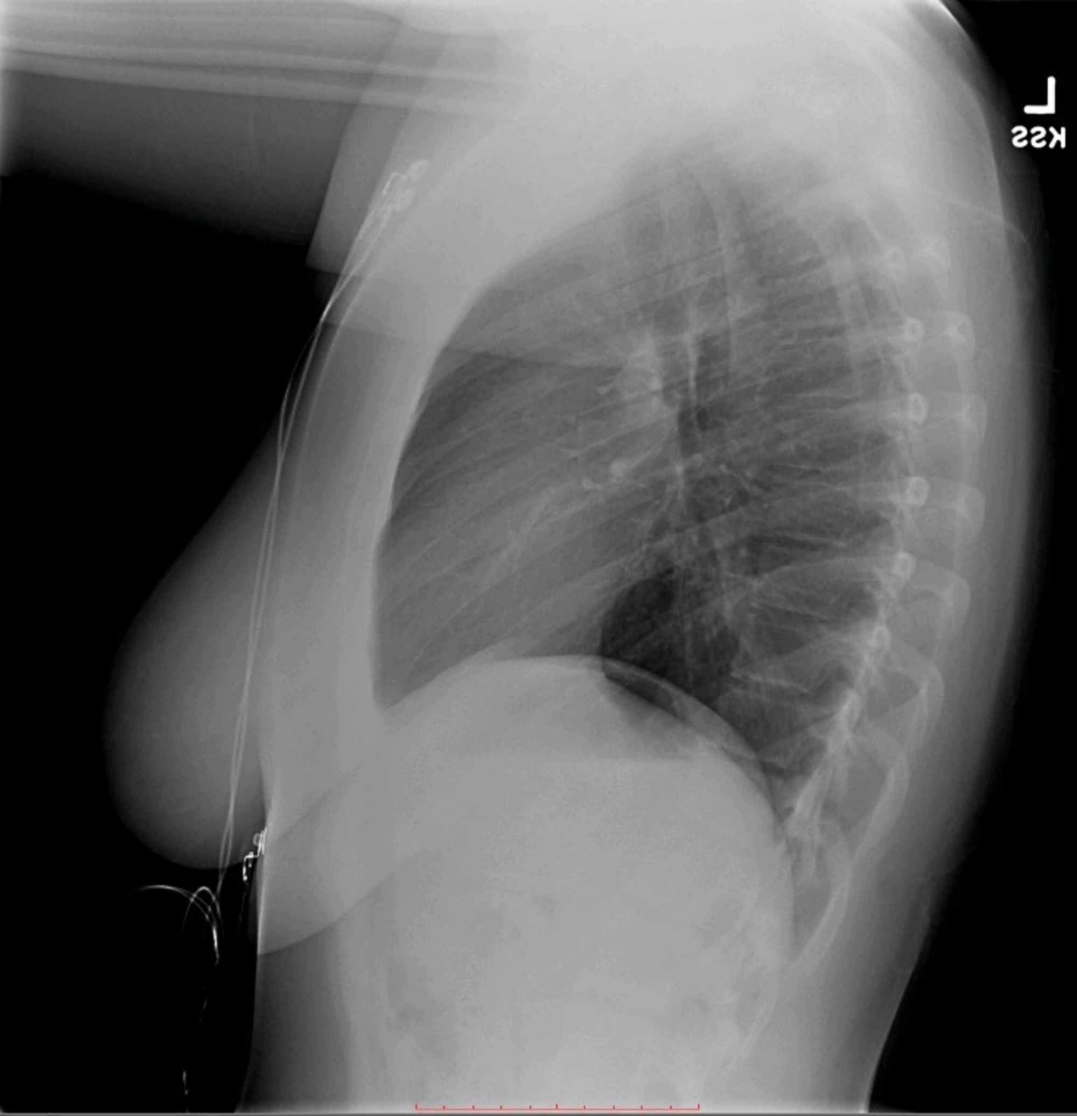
Two-view chest X-ray (lateral view) showing no evidence of acute cardiopulmonary process
